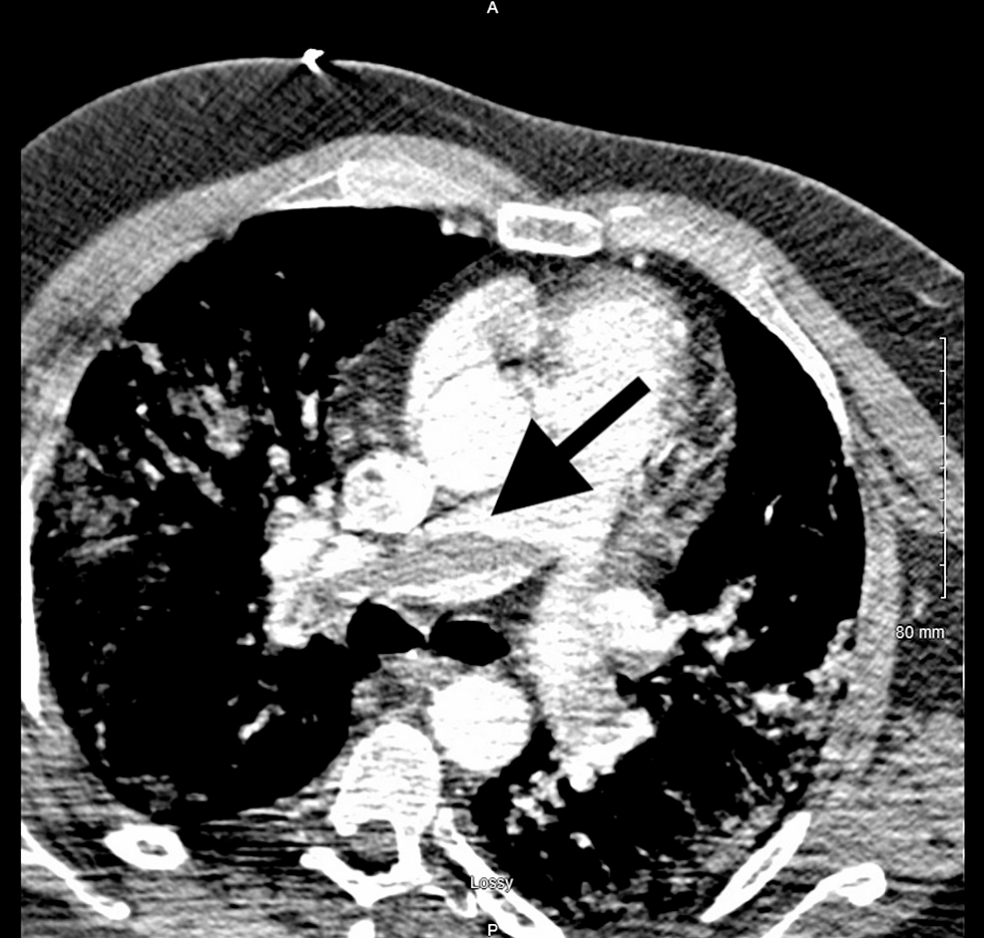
CT showing saddle embolus in the pulmonary artery (arrow) CT: computed tomography
